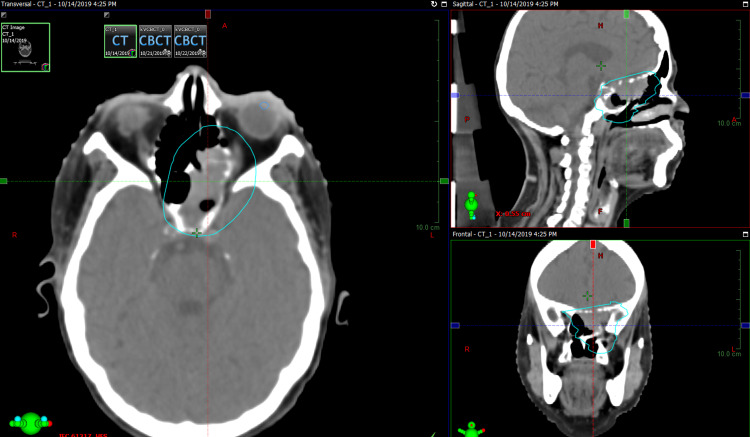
Planning target volume coverage in planning CT scan in all sections (axial, sagittal, coronal; clockwise)
